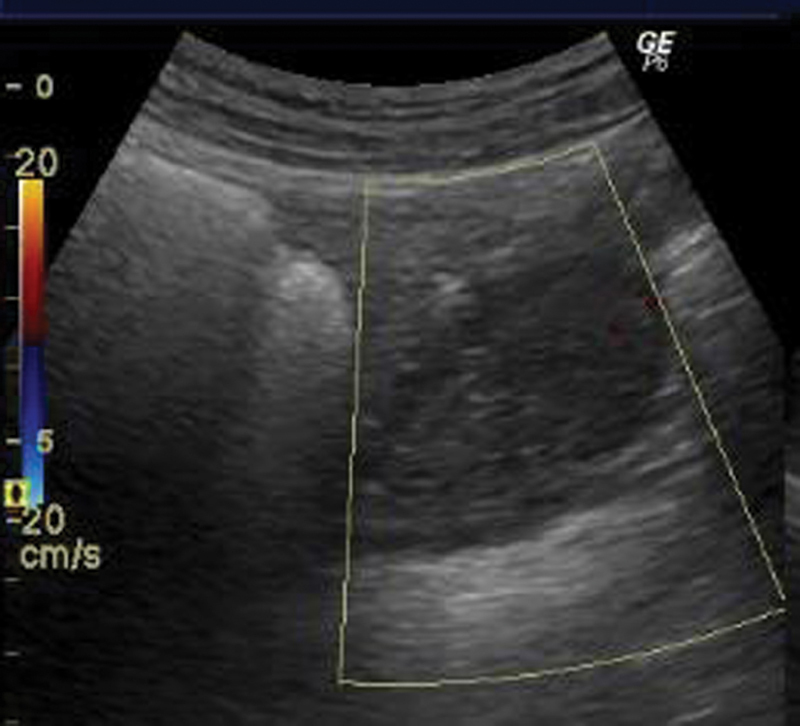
Ultrasound of the right ovary: multiple small follicles in the periphery and no Doppler color sign.
